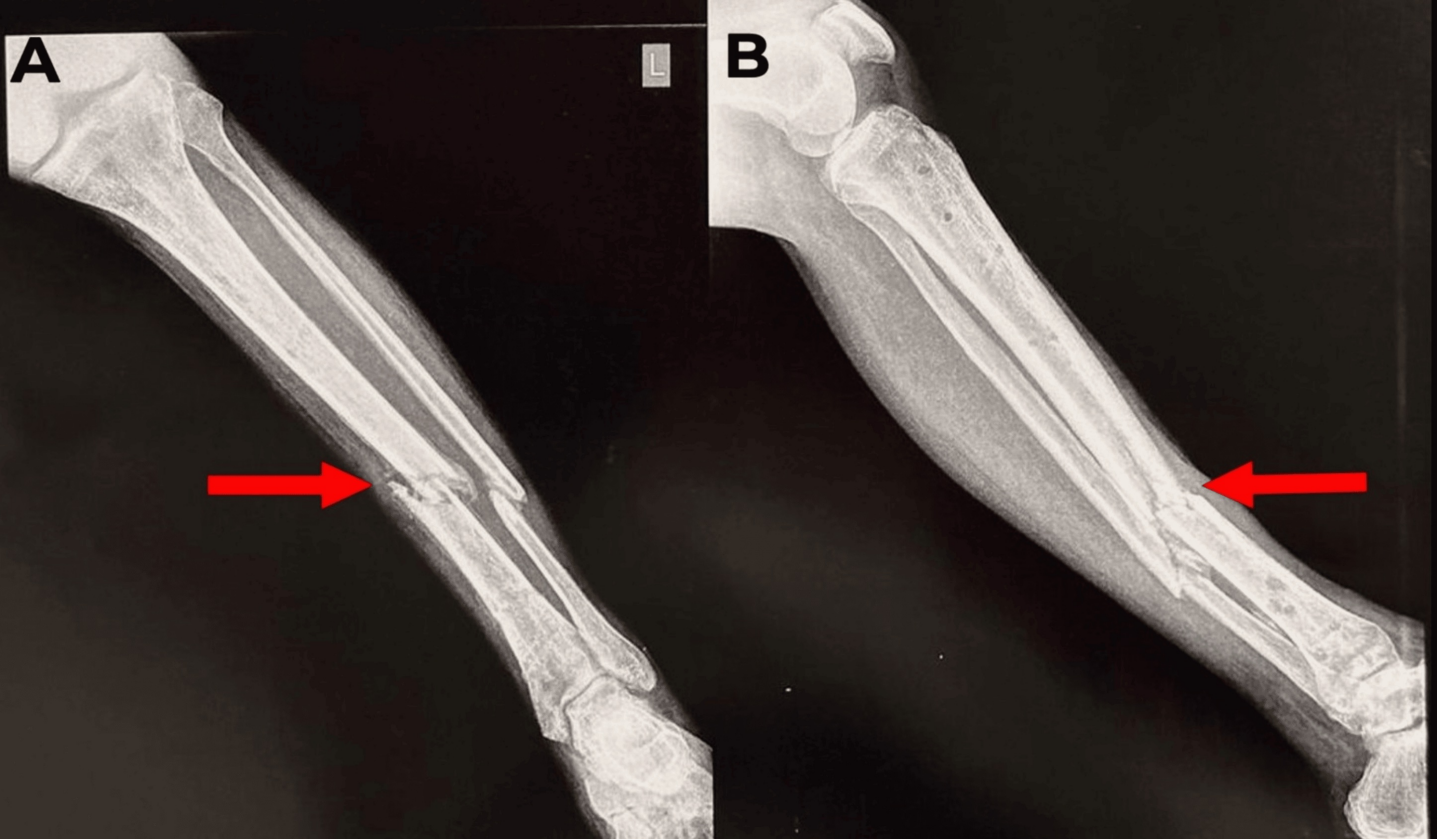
A) the left tibia and fibula X-ray AP view. B) left tibia and fibula X-ray lateral view. Red arrows show non-union of the tibia and fibula mid-shaft fracture. AP: anterior posterior
